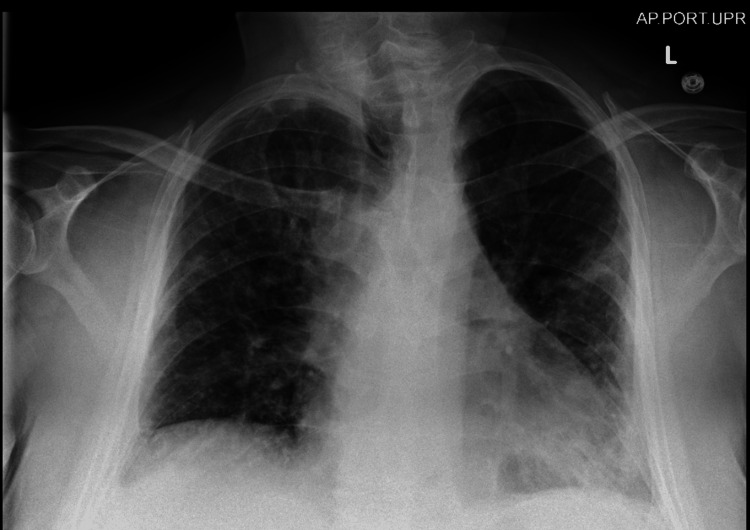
Chest X-ray The cardiomediastinal silhouette is enlarged. There are mild patchy infiltrates throughout the left lung, as well as in the right midlung field. The pleural spaces are clear. The bones and soft tissues are within normal limits
